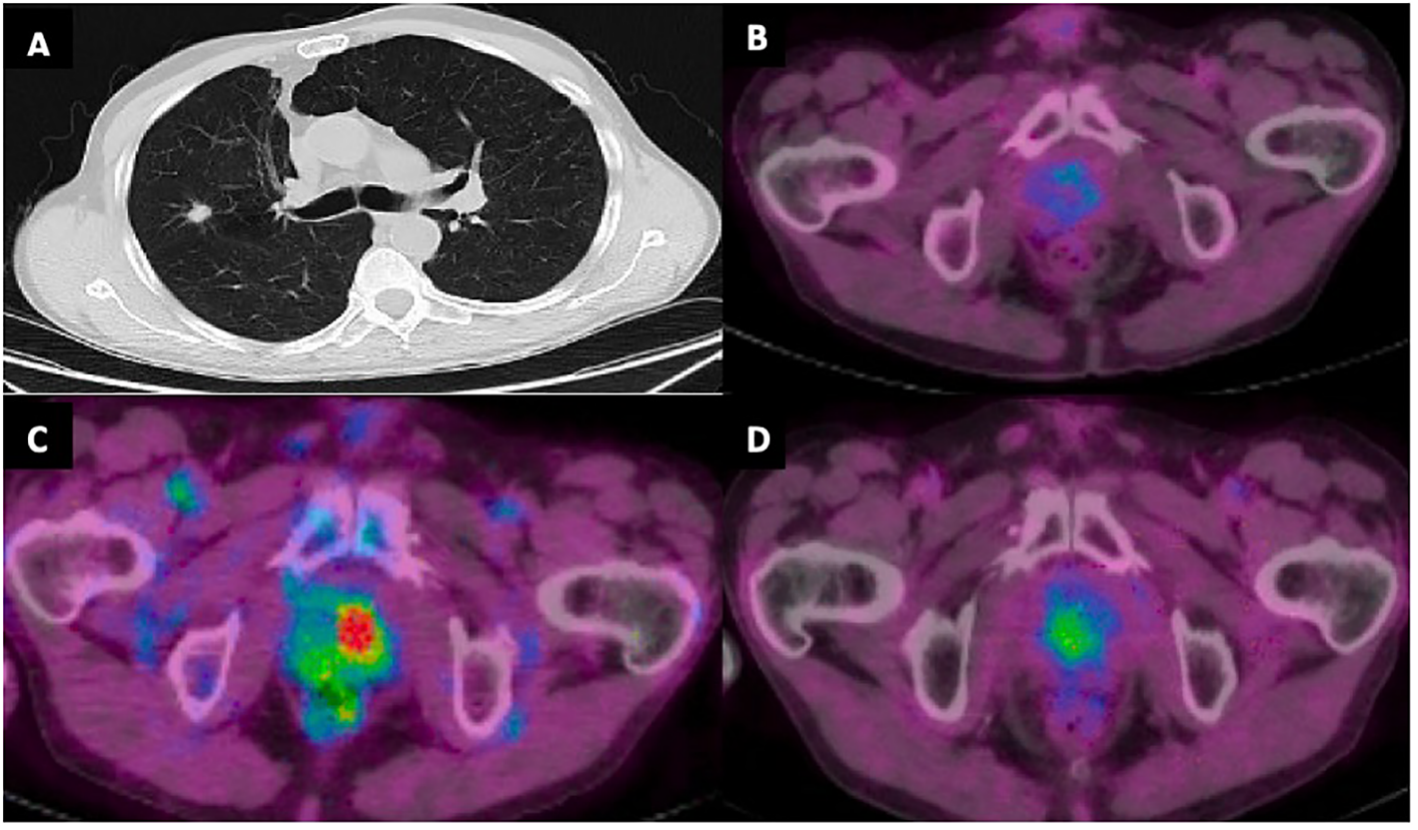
(A) CT chest with 1.4 cm right middle lobe spiculated nodule at time of initial diagnosis. (B) PET/CT demonstrating absence of increased FDG activity in the prostate gland at time of diagnosis. (C) Increased FDG activity in the prostate gland with maximum standardized uptake value (SUV) 8.4 concerning for prostatic metastasis. (D) Decreased FDG activity in prostate gland following SBRT with SUV max 3.6.
